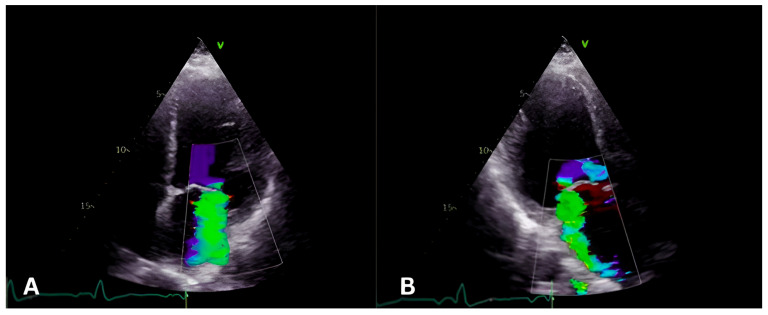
Severe acute mitral regurgitation with color Doppler; (A) apical-4-chamber view; (B) apical-3-chamber view (image courtesy of E. Kiouri).
